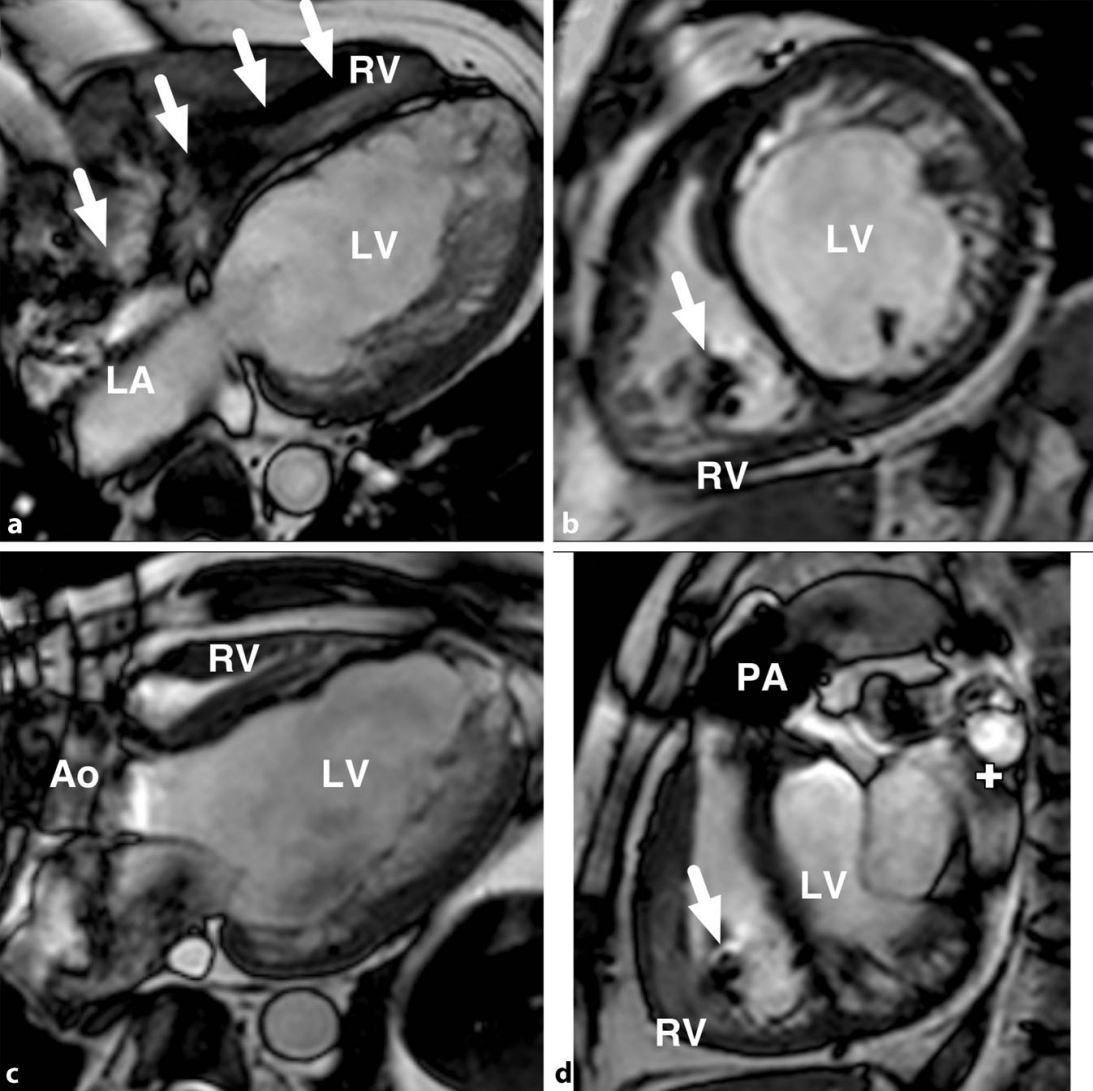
Cardiac magnetic resonance imaging in a patient with congenital aortic stenosis (status post-Ross procedure) and magnetic resonance-conditional implantable cardioverter-defibrillator, demonstrating typical results for balance steady state free precession (b‑SSFP) cine imaging. Lead position is indicated by *white arrows* and the ring artefact related to the generator is seen at the top left of panels (c) and (d). a four chamber view, b short axis, c three chamber view, d right ventricular outflow tract view. *RV* right ventricle, *LV* left ventricle, *Ao* aorta, *LA* left atrium, *PA* pulmonary artery
